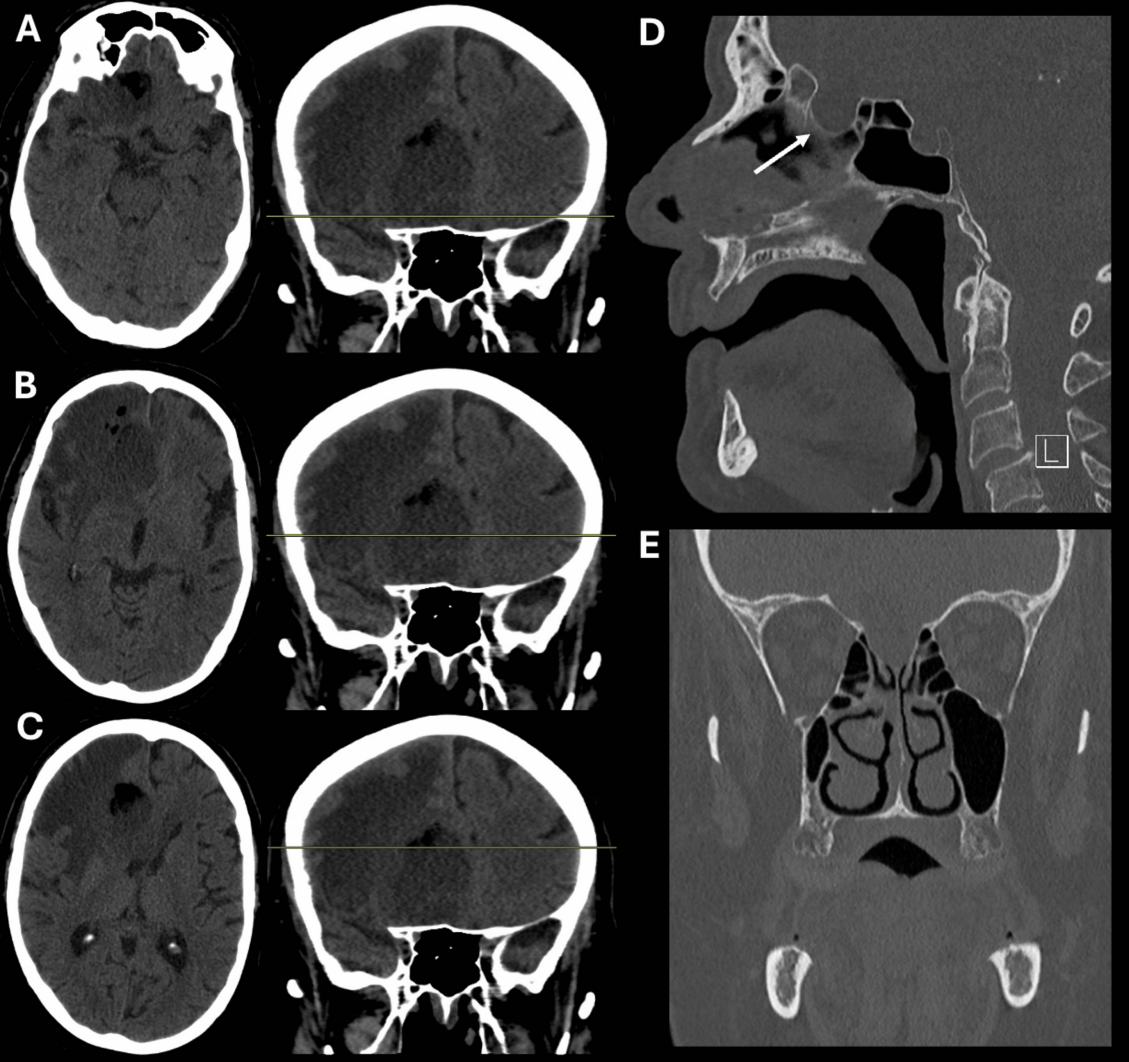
Pre-operative CT scans of head and maxillofacial bones Axial CT head slices, with the level delineated by the yellow line on adjacent coronal images (A-C), demonstrate a mixed-attenuation mass, including fat attenuation (with a CT number of about -29 Hounsfield units), within the inferior medial right frontal lobe, with a large amount of surrounding vasogenic edema and associated local mass effect, resulting in effacement of the anterior aspects of the lateral ventricles (right greater than left) and leftward subfalcine herniation. Sagittal (D) and coronal (E) CT images of the maxillofacial bones demonstrate a well-defined, inferiorly remodeled region of the cribriform plate from just posterior to the crista galli to the planum sphenoidale (white arrow). There is no evidence of dehiscence, destructive bony change, or extension of the tumor into the sinonasal cavity to suggest an aggressive process. CT: Computed tomography
